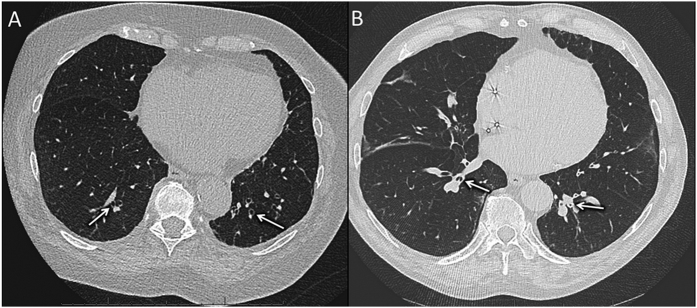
High resolution CT scan. (A) Patient III:6, note mild cylindered bronchiectasis in both lower lobes (white arrows). (B) Patient III:2, there is marked bronchial wall thickening on both sides (whites arrows).
